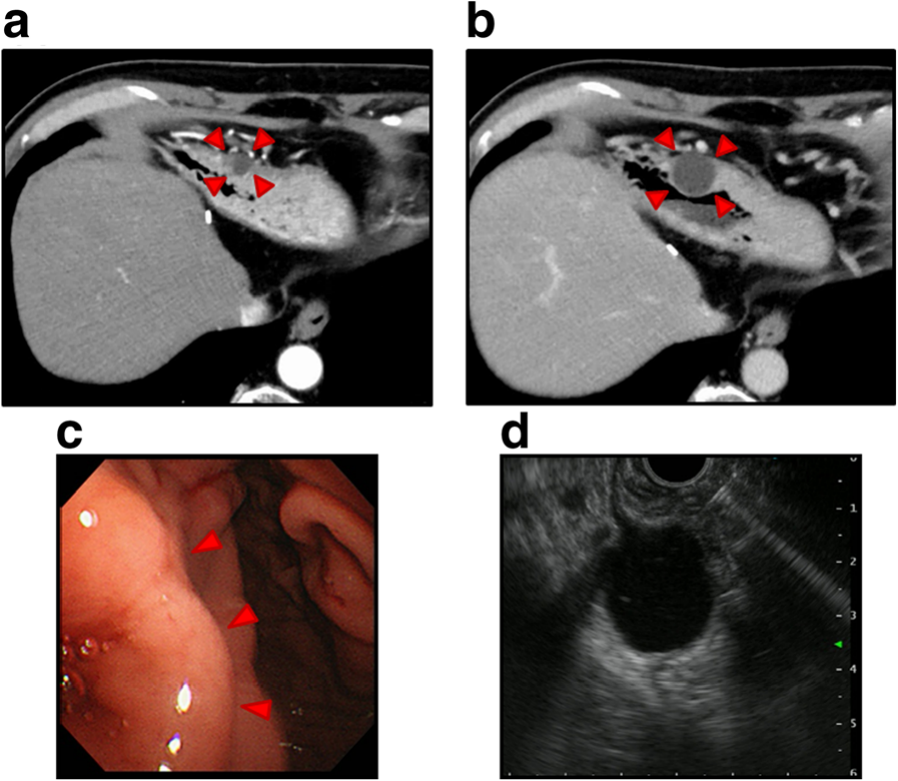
Preoperative imaging of the cystic tumor in the gastric antrum. Enhanced CT showed a 15-mm cystic lesion of the gastric antrum (a, arrowheads) 4 years after the initial operation. Six months later, the cystic lesion had grown up to 25 mm (b, arrowheads). Endoscopy revealed no epithelial lesion (c, arrowheads). Endoscopic ultrasonography showed a submucosal tumor (d)
